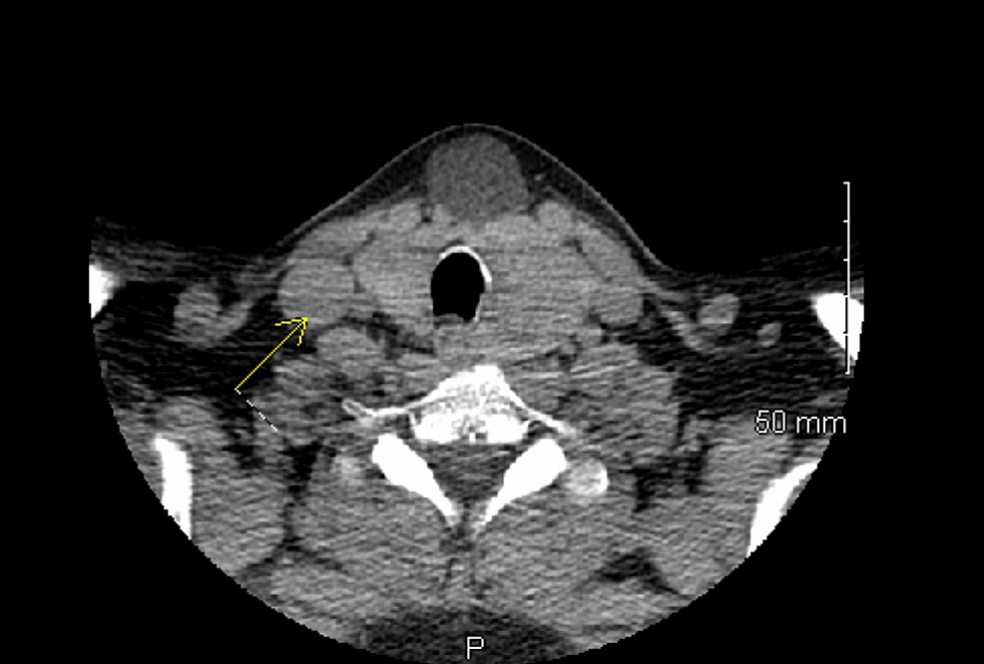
CT without contrast: a thin-walled cystic lesion was located near the thyroid gland.
